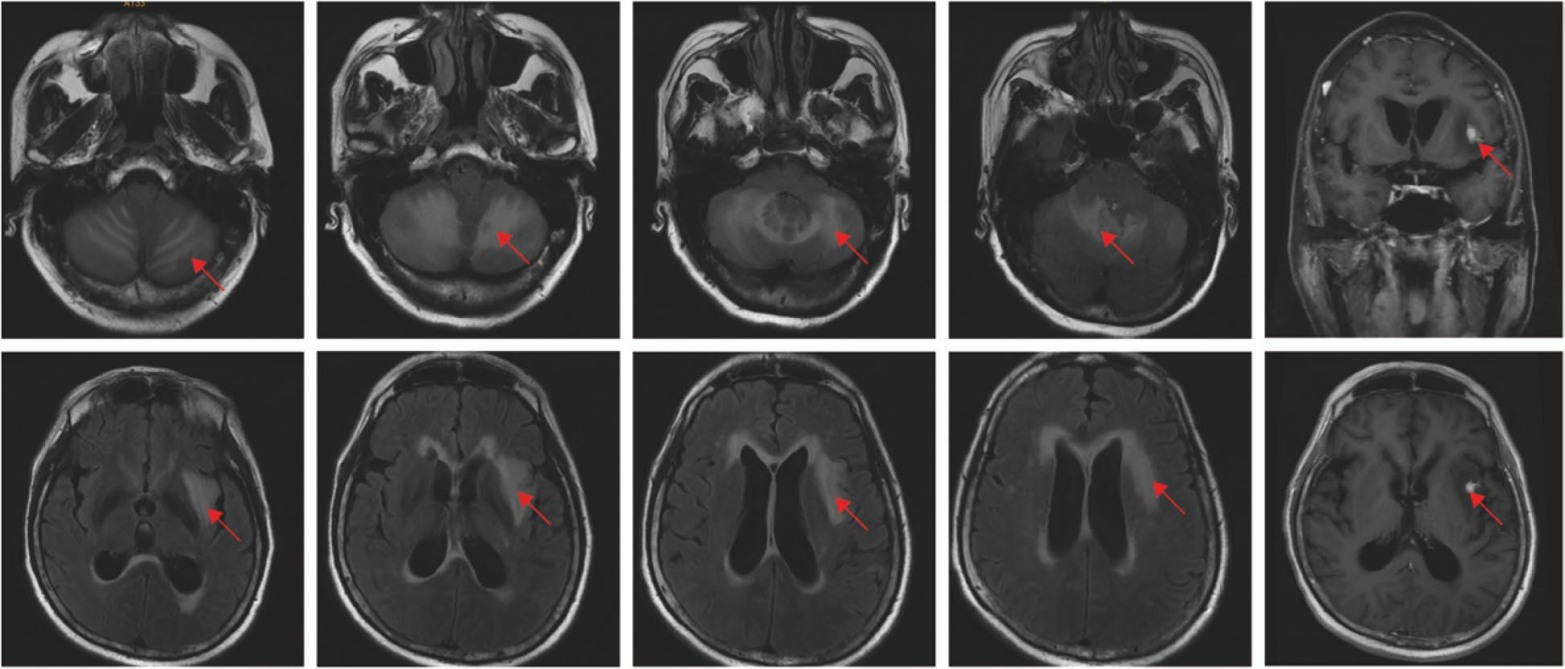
Plain and enhanced head MRI: abnormal signals in left lateral capsule, insular lobe and bilateral cerebellar hemispheres, with significant enhancement in the left insular nodule, left temporal nodule, and bilateral cerebellar meninges. Arrowheads: infection lesions
